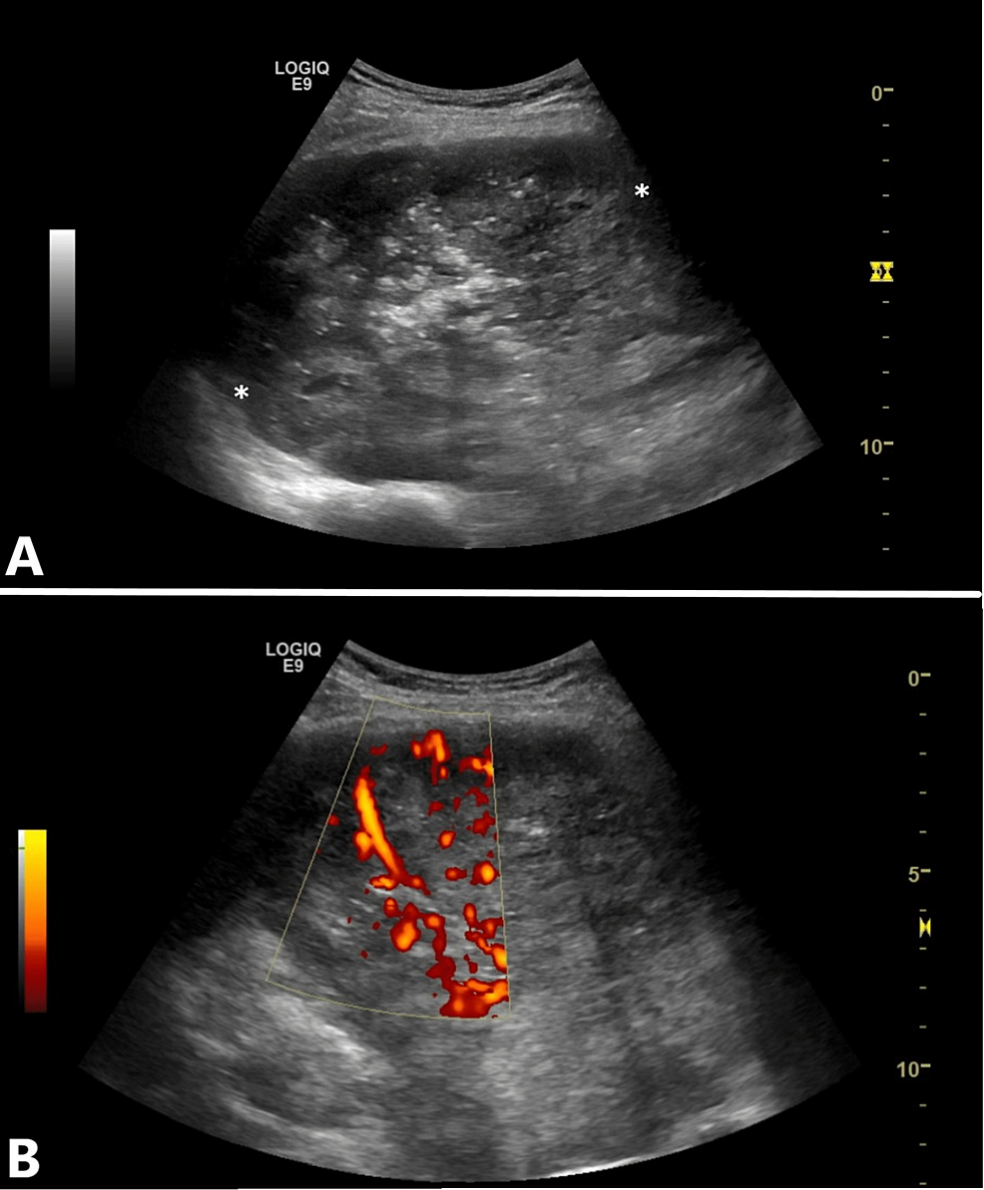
Transthoracic ultrasound A: Grayscale US showing a predominantly hypoechoic mass with amorphous calcifications (asterisks); B: Color Doppler (B-Flow) depicting rich vascularity
